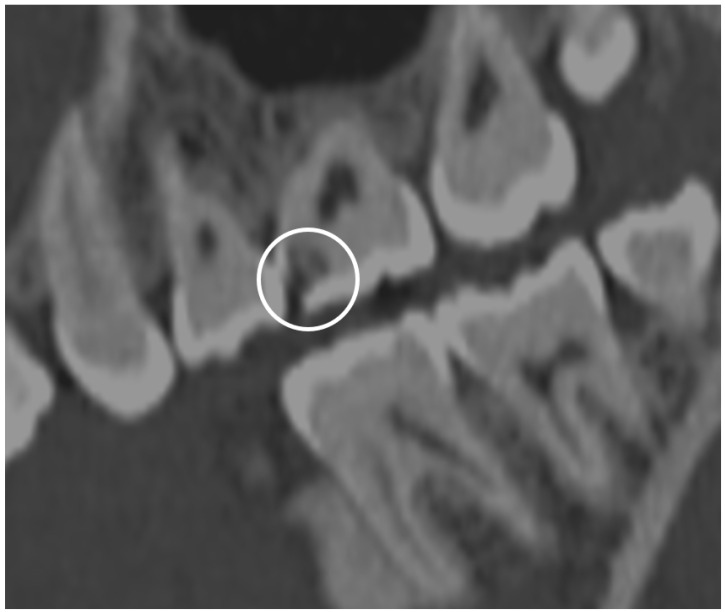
Sagittal section CT demonstrating lucency (white circle) within the enamel and dentin layer of a maxillary first molar, compatible with decay.
